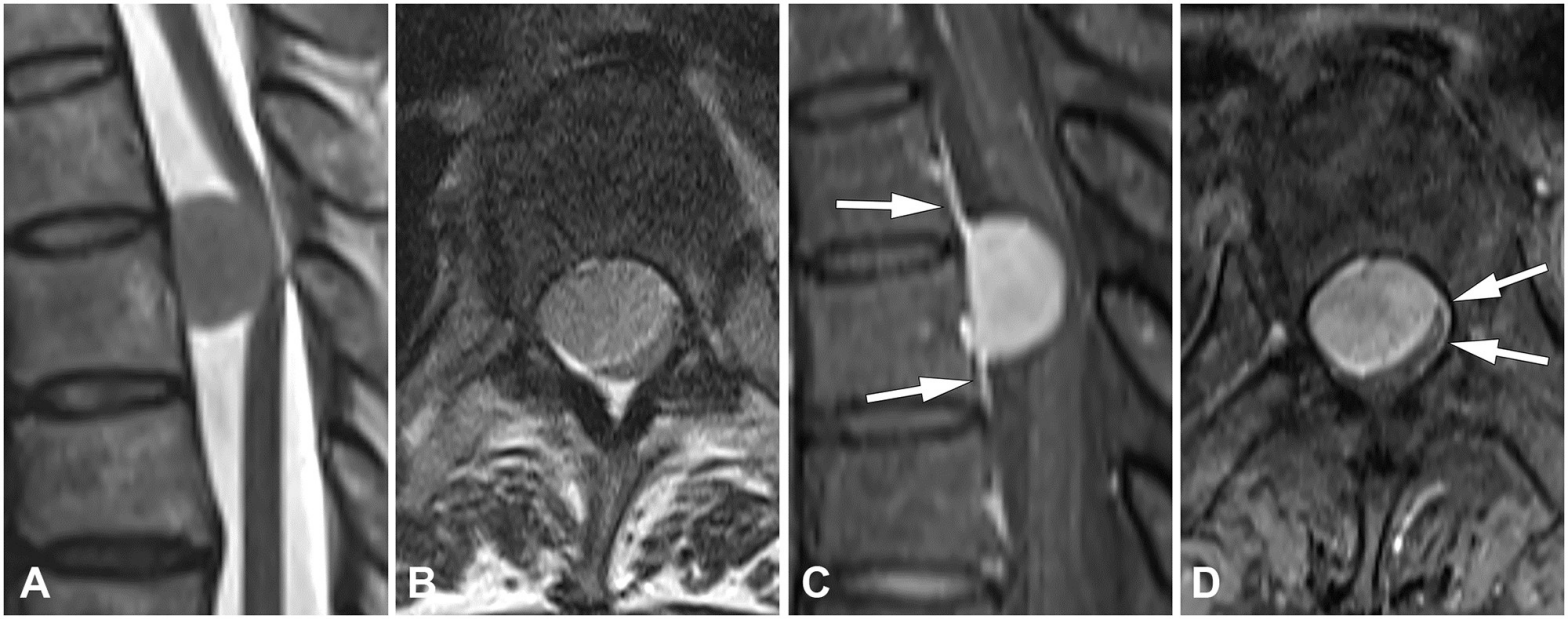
A meningioma in a 53-year-old woman. (A) Sagittal and (B) axial T2-weighted images show an intradural extramedullary mass on the T12 level that is slightly hyperintense than the spinal cord (C) Sagittal and (D) axial fat-suppressed contrast-enhanced T1-weighted images show thickening with enhancement of dura mater adjacent to the tumor that tapers away from it, representing dural tail sign (arrows).
